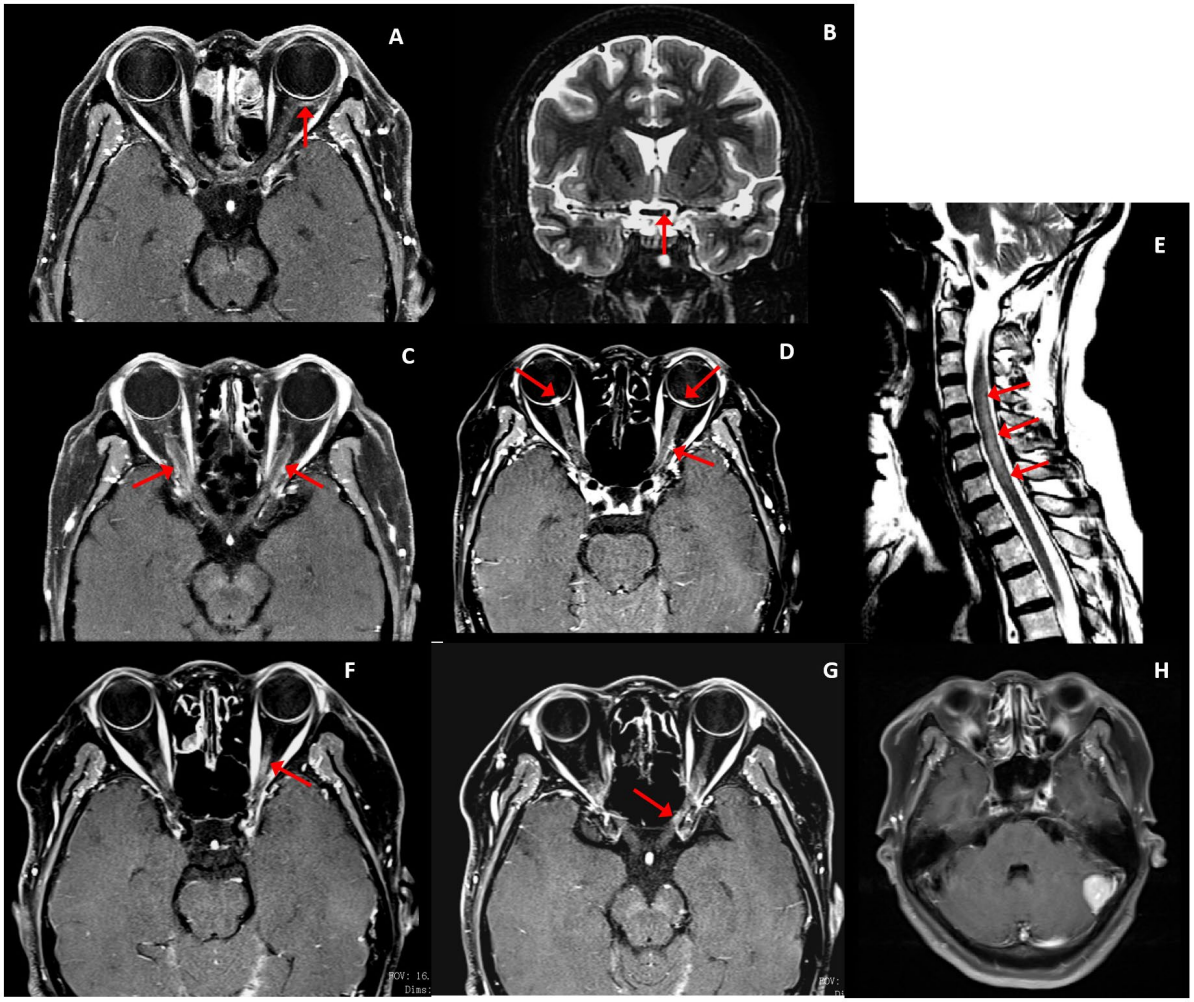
Optic nerve and spinal cord MRI (red arrowed). Patient 1 (F, 59 years): (A) Post-gadolinium axial T1 MRI demonstrated left bulbar fascia mild enhancement. (B) T2 lesion on the left side of the optic chiasma. Patient 4 (F, 55 years): (C) Post-gadolinium axial T1 MRI demonstrated bilateral optic nerve sheath mild enhancement. Patient 3 (F,53 years): (D) Bilateral optic nerve sheath mild enhancement and optic disk enhancement. (E) C2–7 T2 lesions. Patient 5 (F,61 years): (F,G) Left optic nerve enhancement. (H) Left cerebellar meningioma.
